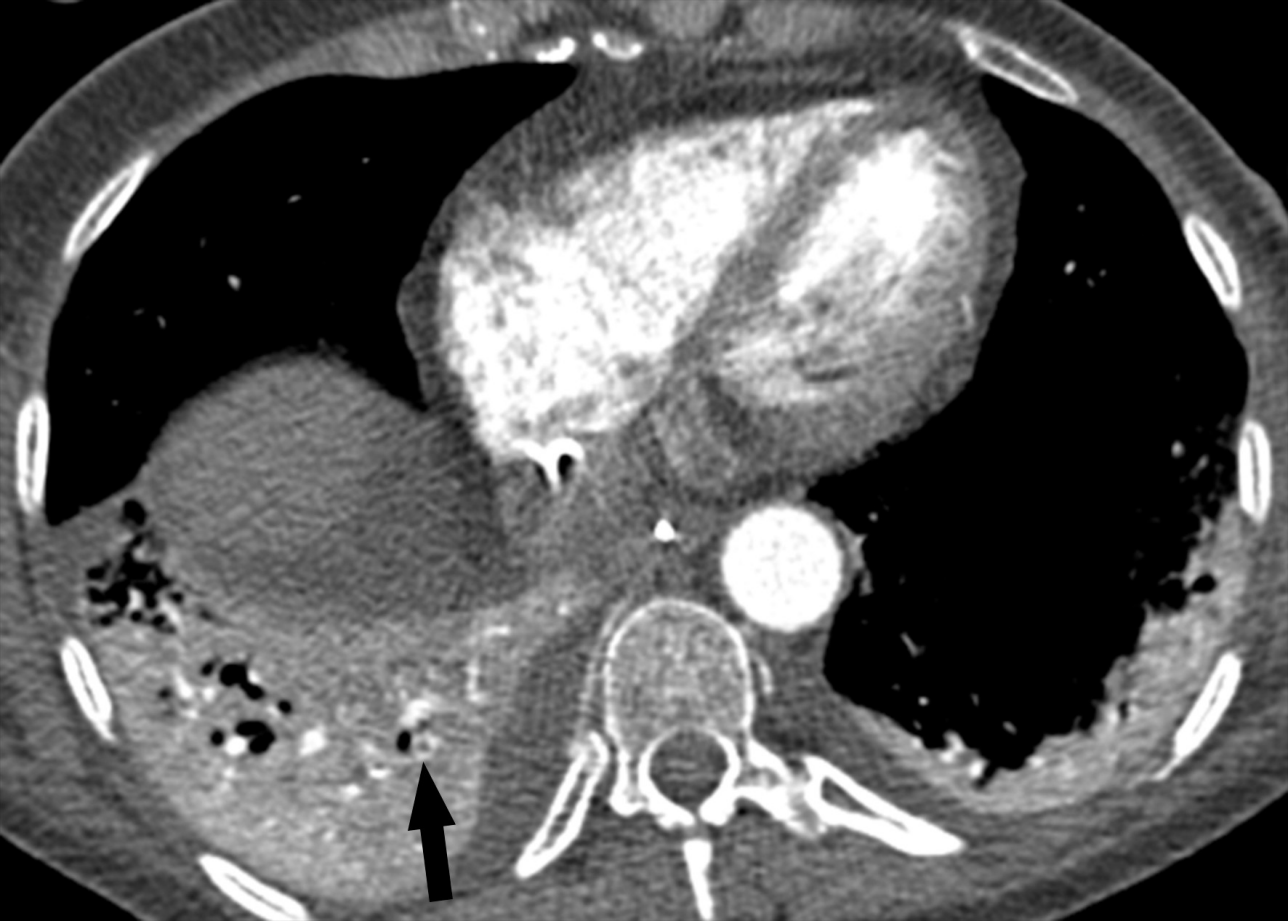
Pulmonary embolism. Axial reconstruction of a CT in soft tissue window showing pulmonary embolism of the right lower lobe.
